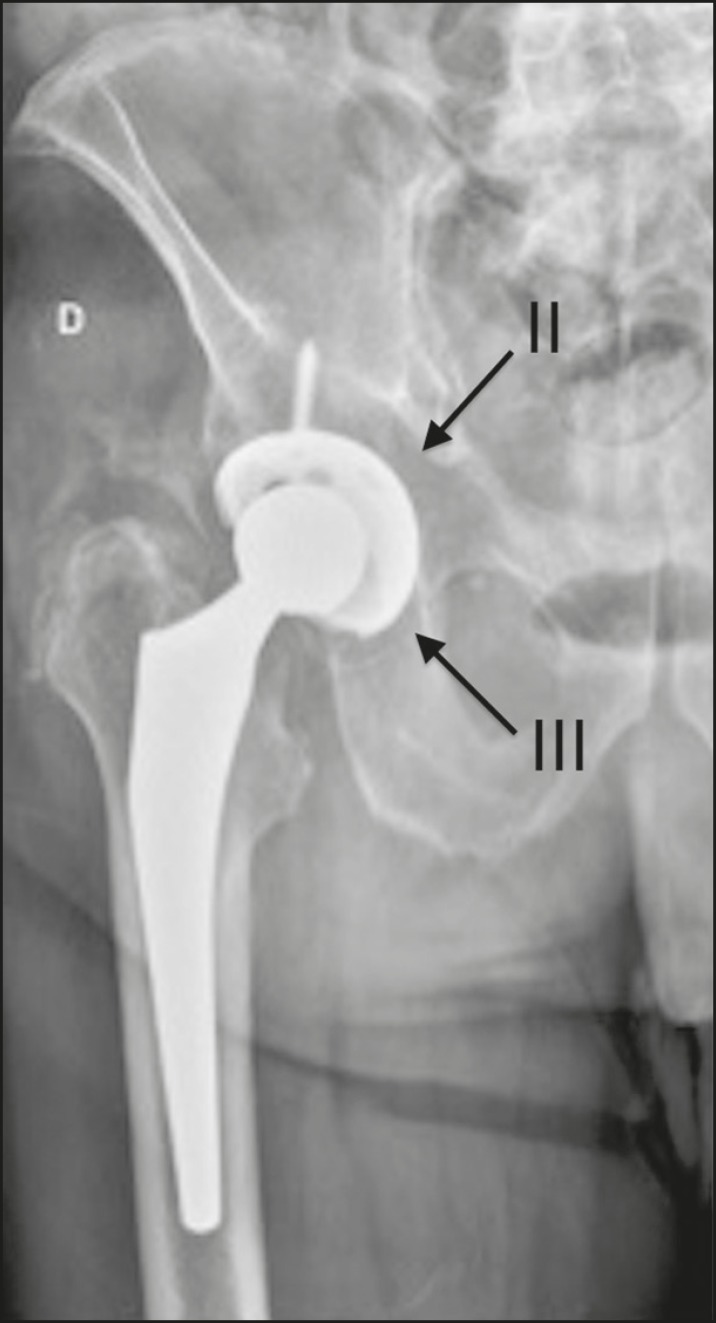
Anteroposterior X-ray of the right hip, showing osteolysis in acetabular zones II and III (arrows).
